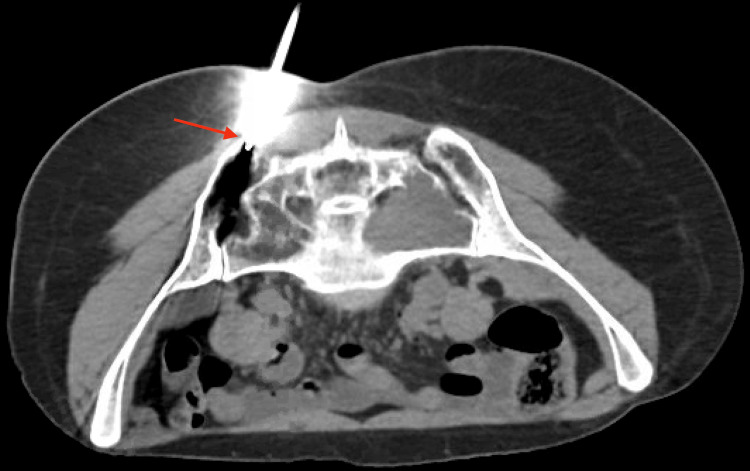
Red arrow shows CT-guided bone marrow biopsy and aspiration. CT: computed tomography
